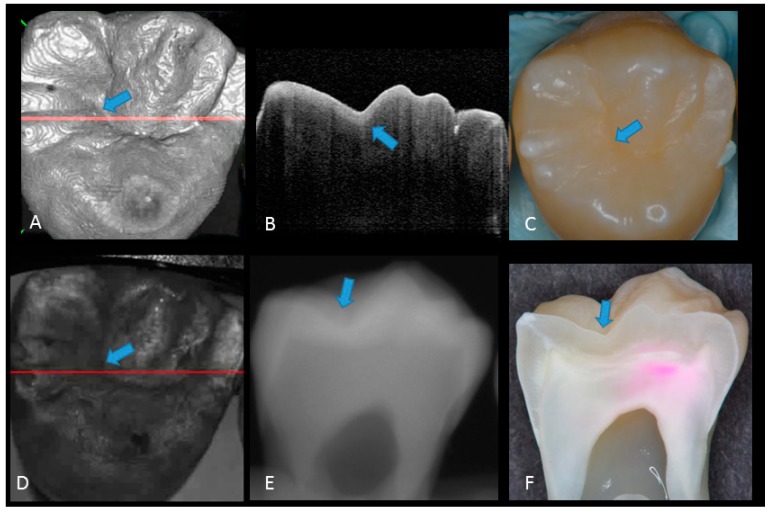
Sound enamel. (A) gray-scale three-dimensional (3D) OCT image; (B) cross-sectional OCT image along the red line in (A) and (D) with intact enamel with bright band across the fissure base extending over the cusps (arrow), suggesting a developmental feature (not acquired caries); (C) photographic presentation of occlusal enamel with seemingly intact fissure (arrow); (D) OCT en face intensity projection; (E) radiograph; (F) cross-sectional photograph with no dye at the observed fissure (arrow) confirming sound surface (score 0). The dynamic slicing 3D video is presented in Supplementary Materials: Video 1.
